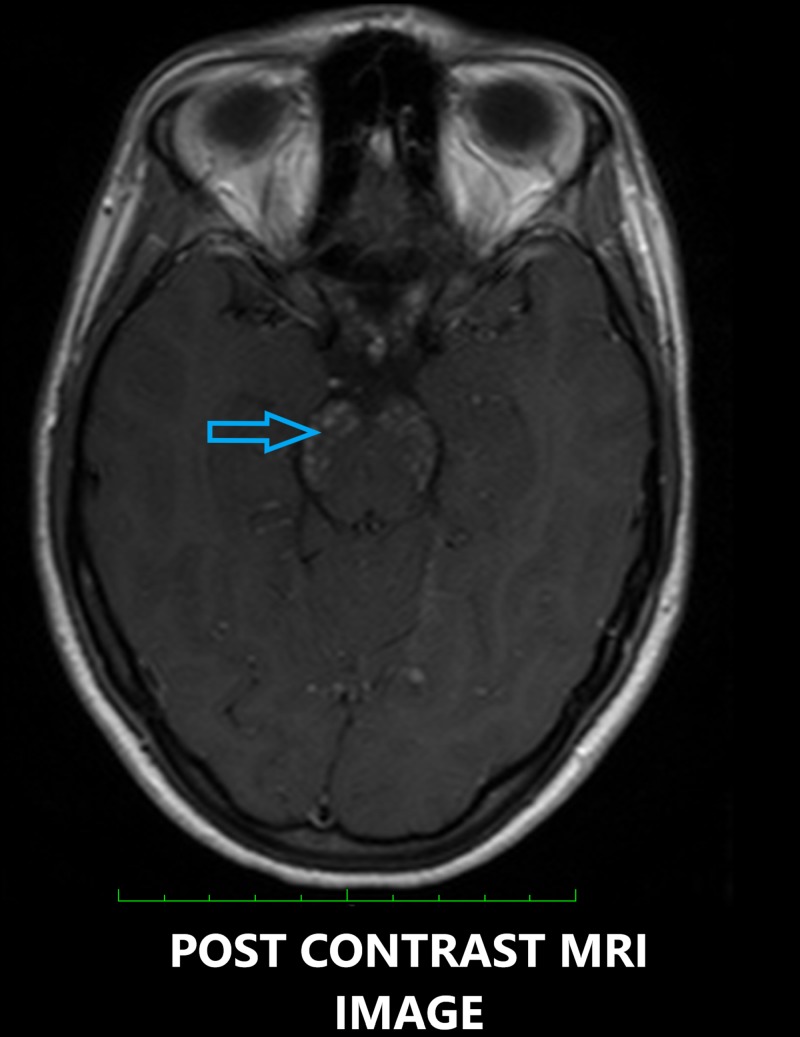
Contrast-enhanced axial magnetic resonance imaging (MRI) showing peppered post-contrast enhancement in the pons (blue arrow).
